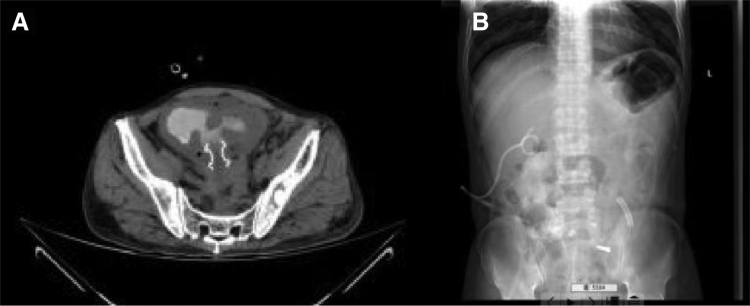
LAMS stent position is good, and postoperative symptoms are relieved. A, CT confirms the position of the LAMS stent. B, Abdominal plain film clearly shows obstruction relief. CT, computed tomography; LAMS, lumen-apposing metal stent.
